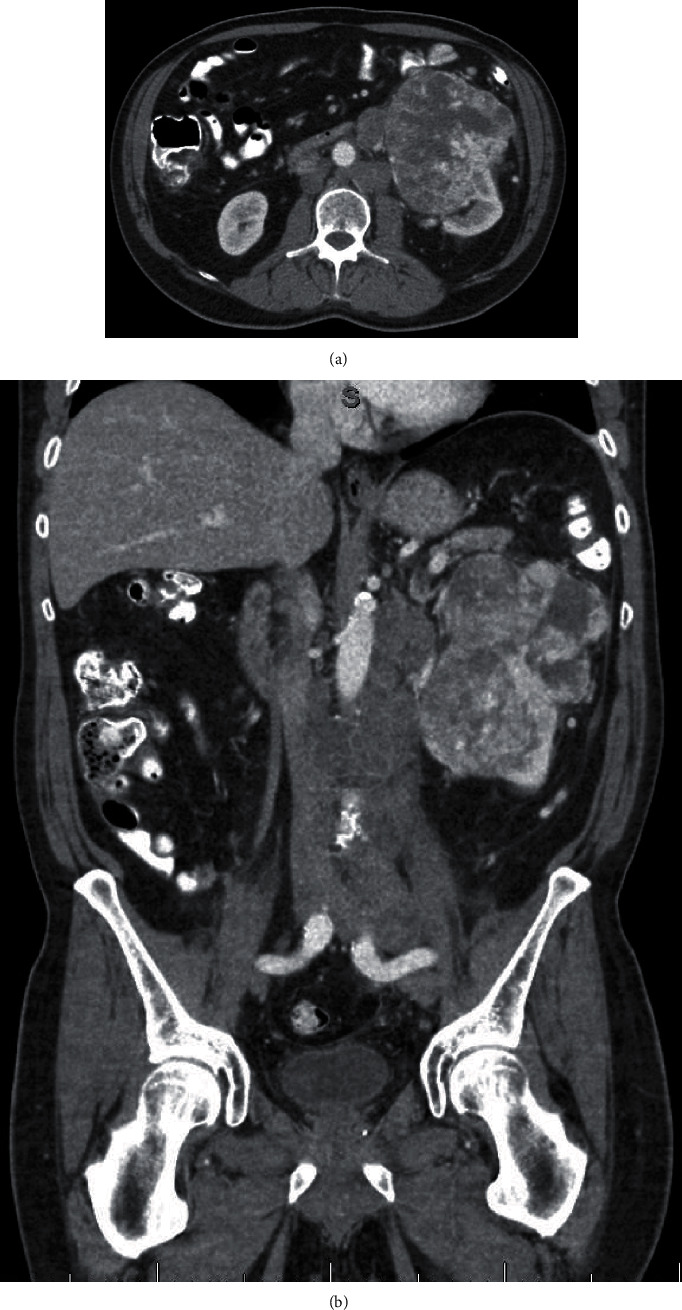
CT imaging prior to nivolumab/ipilimumab treatment: (a) axial imaging demonstrating the heterogeneously enhancing left renal mass; (b) coronal imaging demonstrating the enlarged retroperitoneal lymphadenopathy.
